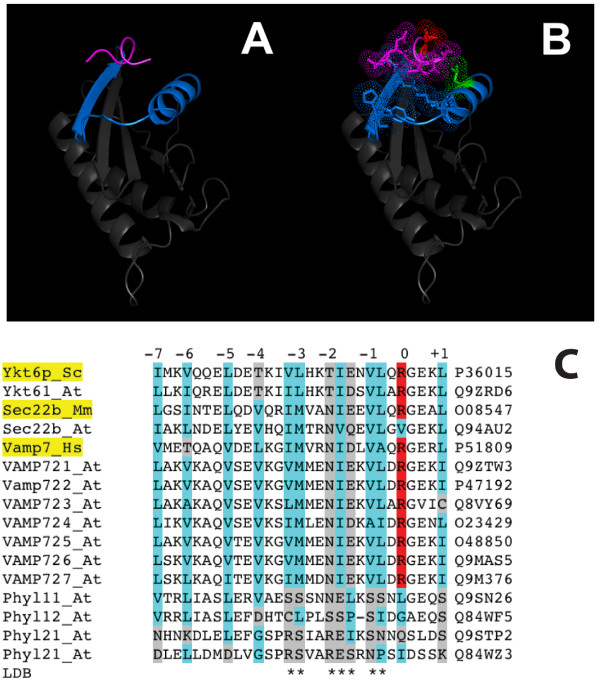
Phyre (threading method) based prediction of intramolecular binding in a representative Phytolongin [UniProt: Q9SN26]. Panel A: a short motif from the PhyL region (magenta) is suggested to bind to the α1-β3 region (blue) of the LD (grey). Panel B: binding is likely based on polar side chains (LD, blue; PhyL motif, magenta); hydrophobic side chains from the LD are green and the only one from the PhyL region is red. Structural representations were obtained using PyMOL. The alignment in panel C is centered around the LD binding (LDB) motif of structurally solved longins (highlighted in yellow). Homologous Ykt6, Sec22b and VAMP7 family longins and the four Phytolongins from *Arabidopsis thaliana *are also included. The conserved Arg residue at the zero layer of the SNARE motif is highlighted in red. Hydrophobic or polar residues are highlighted in respectively cyan and grey in columns concerning the heptad repeat layers or the LDB. The putative LDB region of Phytolongins is clearly more polar than LDB of longins, and the Arg residue is not conserved. Instead, several hydrophobic layer positions are conserved with the Phytolongins. Conservation is not apparent in the CT half (not shown).
